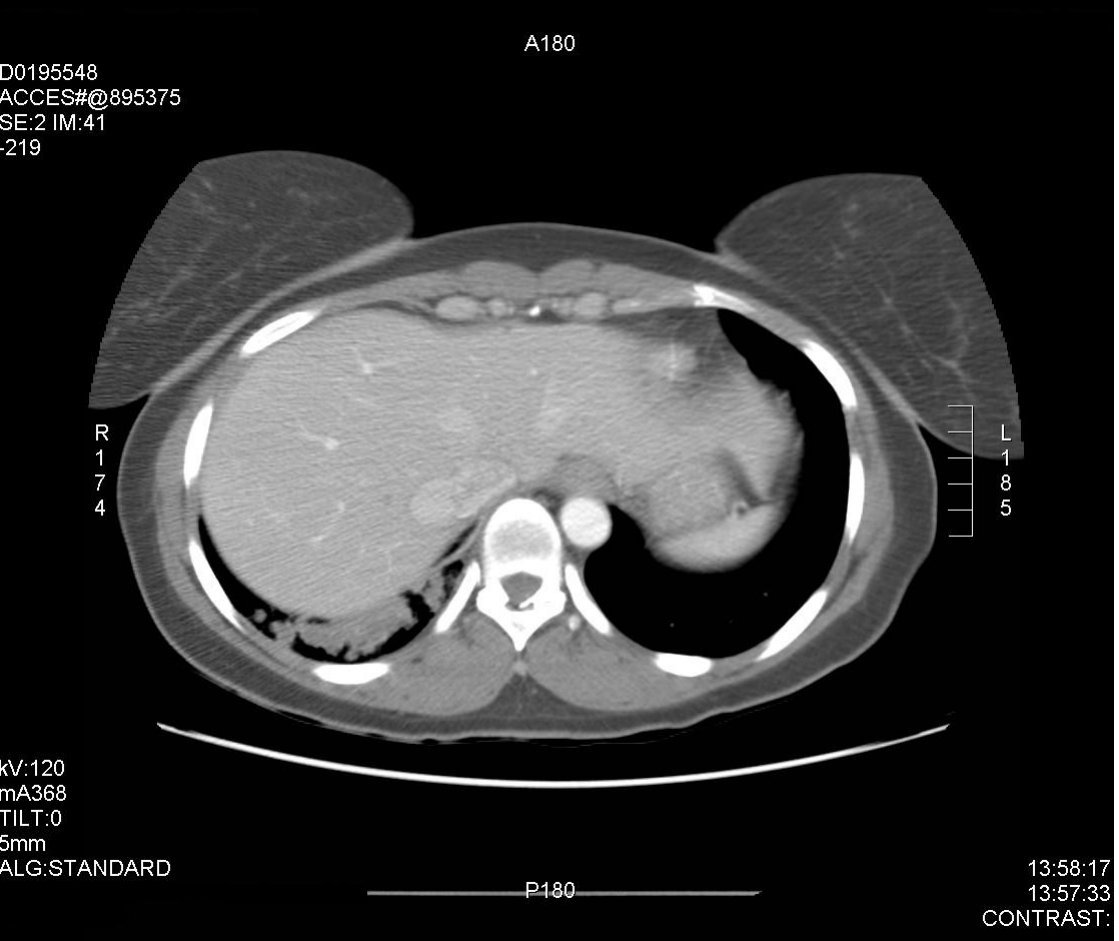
CT scan of the lower lungs/upper abdomen indicating the aberrant feeder artery originating from the descending aorta to the area of the right lower lobe mass.
